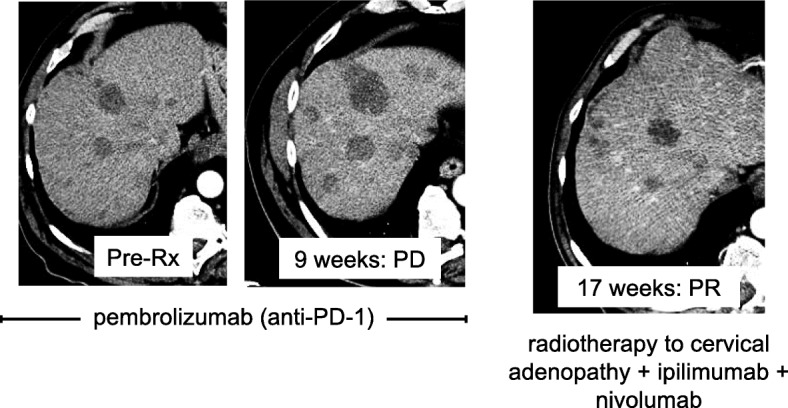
Representative CT images from a 79-year-old man with advanced Merkel cell carcinoma. He experienced progressive disease after receiving pembrolizumab (two left panels). He then received ipilimumab + nivolumab + radiotherapy to cervical adenopathy, to which he developed a partial response (RECIST v1.1) 17 weeks into combinatorial therapy (right panel). PR was ongoing at 8 months when the patient died from complications related to encephalopathy, likely immune-mediated (i.e., a toxicity related to immune checkpoint inhibitor therapy)
